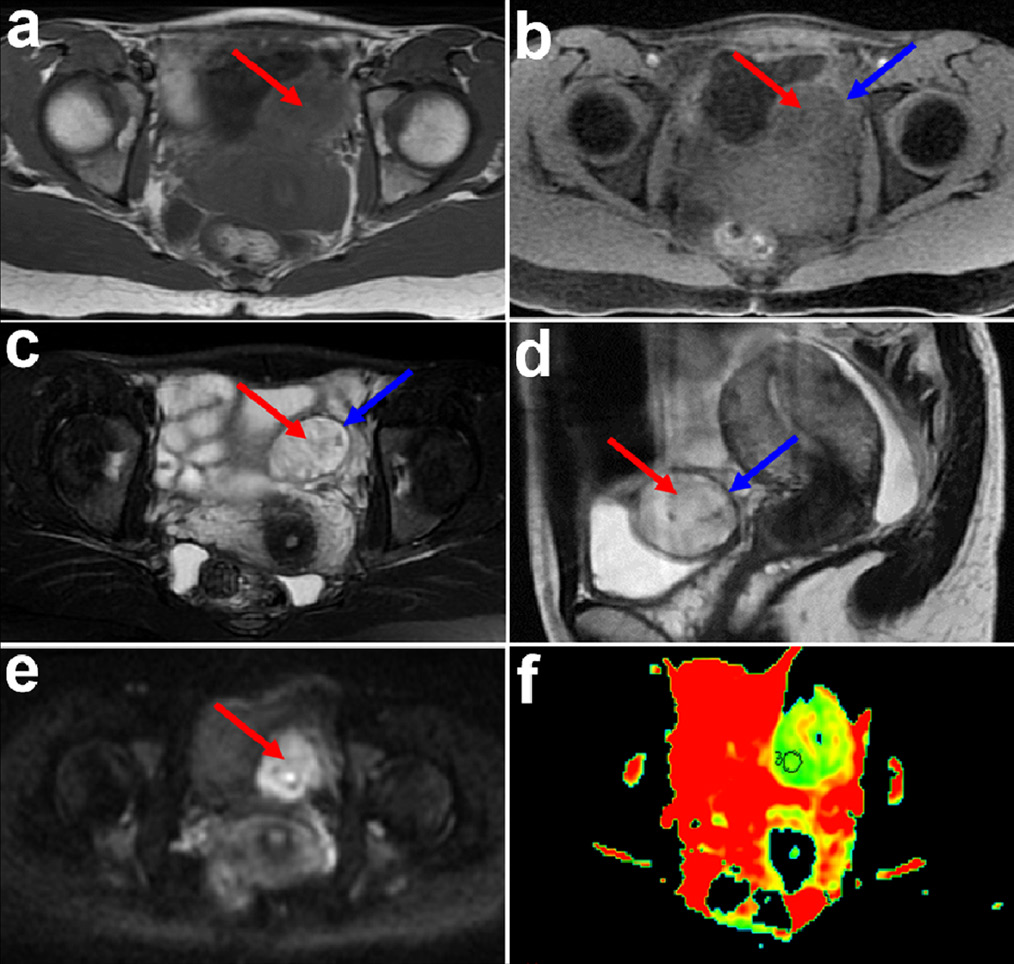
MRI and DWI of the IMT locating in the bladder. (a) Axial T1WI shows a well-defined, oval-shaped extravesical mass (58 × 25×13 mm) adhering to the left posterior bladder wall and protruding through the bladder lumen with slight hyperintensity compared to muscle (red arrow). (b) Fat-saturated axial T1WI exhibits hypointensity mass (red arrow) with isointensity capsule (blue arrow) compared to muscle. (c, d) Axial T2WI with fat-suppression(c) and sagittal *T*
